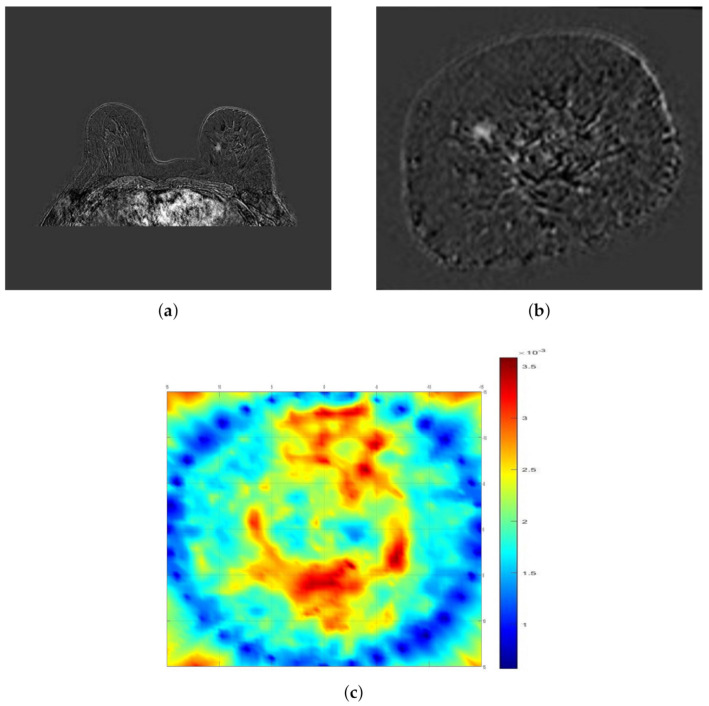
Comparison of SAFE image with clinical MRI: (a,b) MRI scans showing malignant lesion in the left breast (axial and coronal planes) and (c) SAFE scan showing no concordance with MRI (coronal plane) because of air gaps.
